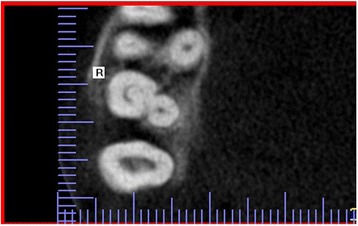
Cross-sectional CBCT image showing C-shaped canal configuration in maxillary second molar
